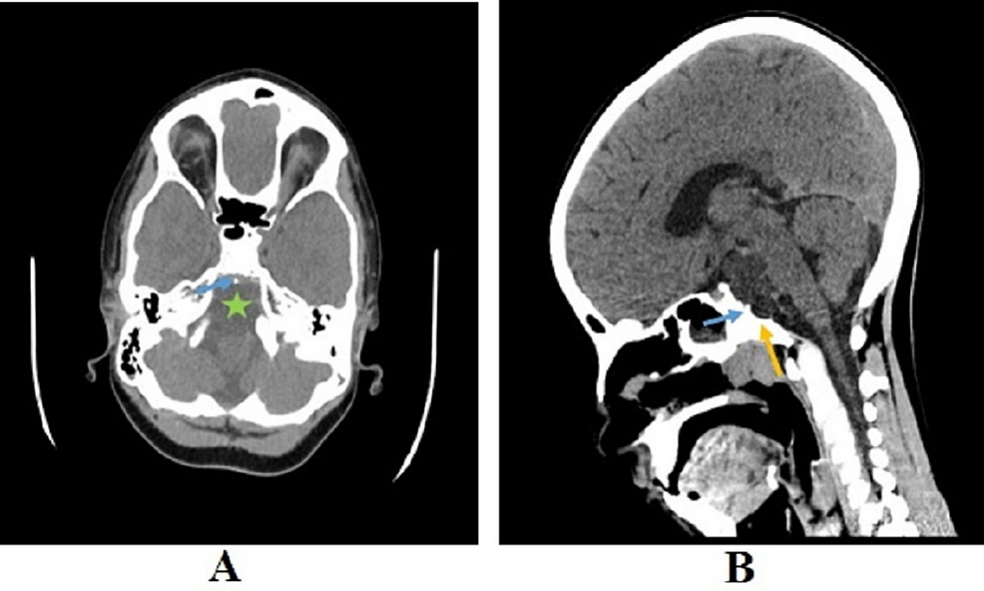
CT scans of the patient CT scans of the patient show (A) hypodense lesion in the prepontine cistern, without calcification (green star), osseous stalk (blue arrow; axial soft tissue window CT image), and (B) smooth scalloping of the posterior clivus (orange arrow) and osseous stalk (blue arrow; sagittal unenhanced CT image).
